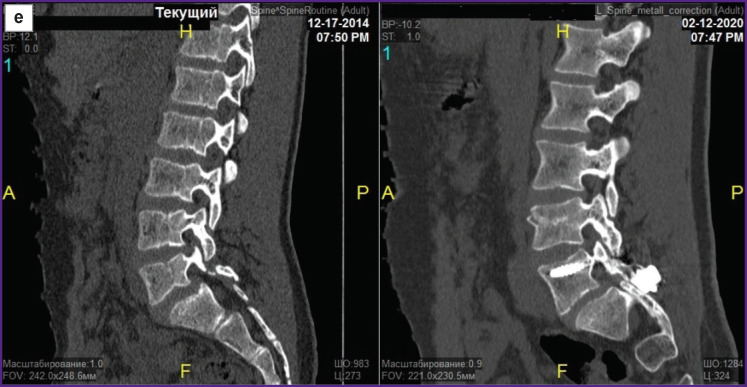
Clinical example of treating spondylolisthesis using minimally invasive technique: (а) minimally invasive dorsal access at the level of the L_5_ vertebra; (b) axial computed tomography: left — preoperative, shows the defect at the level of the L_5_ vertebra; right — postoperative, shows transpedicular screws in the arch of the L_5_ vertebra; (c) radiograph in the direct anterior projection: left — preoperative, shows the defect at the level of the L_5_ vertebra; right — postoperative, shows defect fixation with transpedicular screws at the level of the L_5_ vertebra; (d) radiograph in the lateral projection: left — preoperative, shows the defect at the level of the L_5_ vertebra; right — postoperative, defect was removed using pedicle-screw and hook constructs; (e) lateral CT: left — preoperative, shows the defect at the level of the L_5_ vertebra; right — postoperative — shows two-sided screws on the pedicle and rod and hook constructs on the L_5_ vertebra for defect correction
